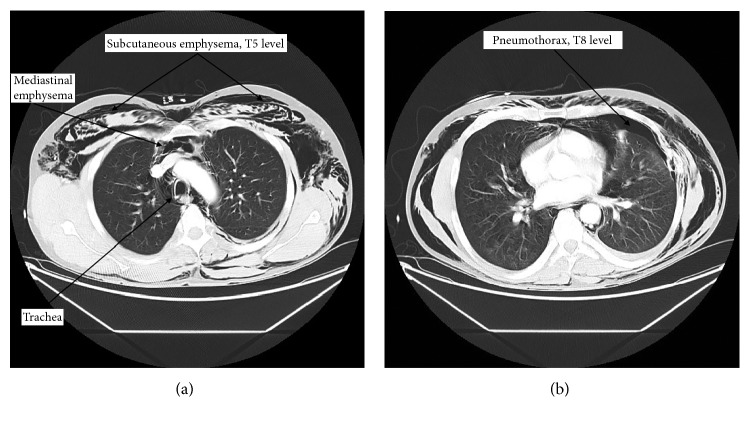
(a) Axial CT image at the T5 level demonstrating extensive subcutaneous and mediastinal emphysema. (b) Axial CT image at the T8 level demonstrating a pneumothorax on the left side. Extensive bilateral subcutaneous air is also visible.
